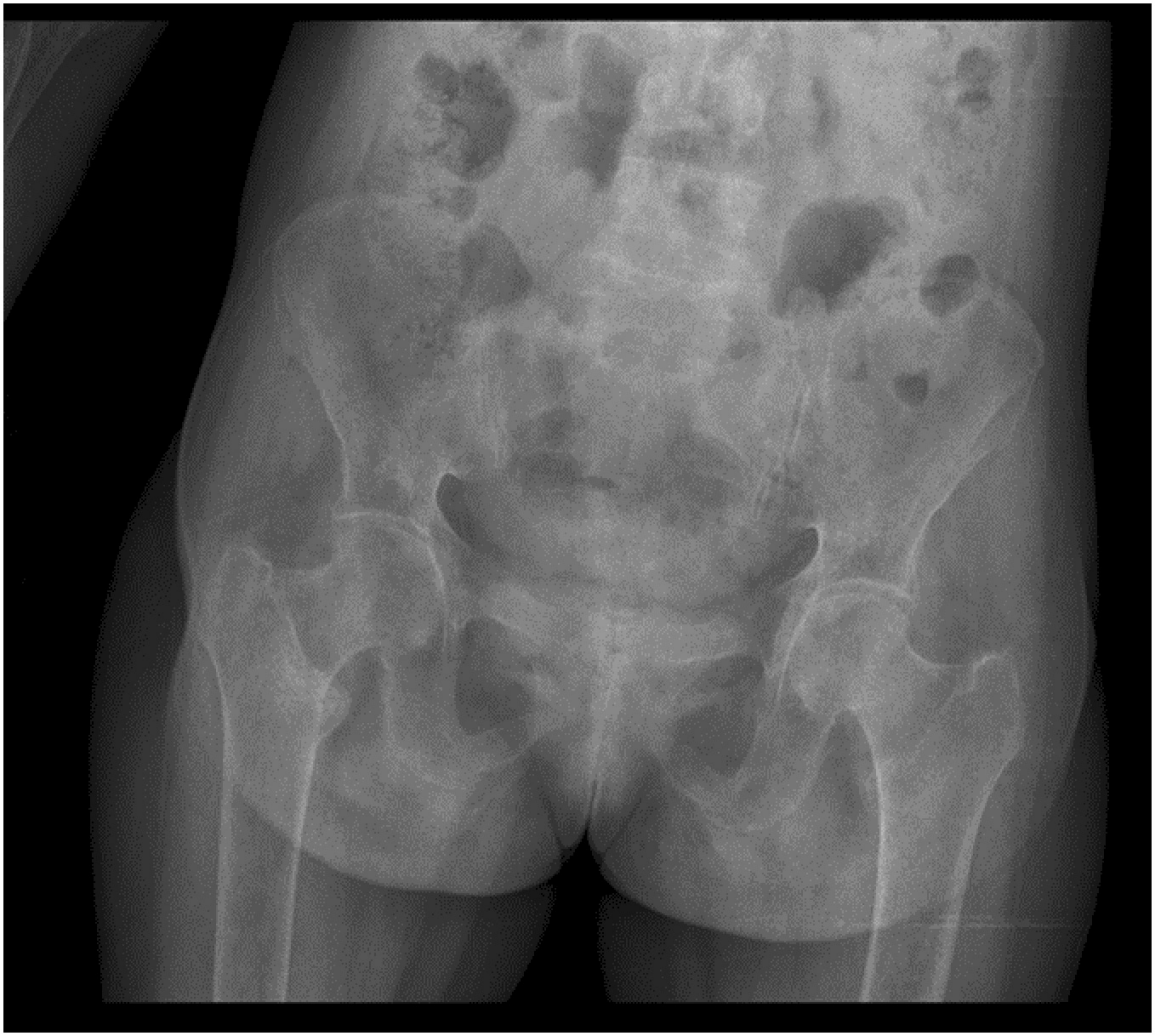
Pelvic radiograph reveals thinning of the cortical bone and loss of trabeculae especially at the proximal aspect of the femur.
